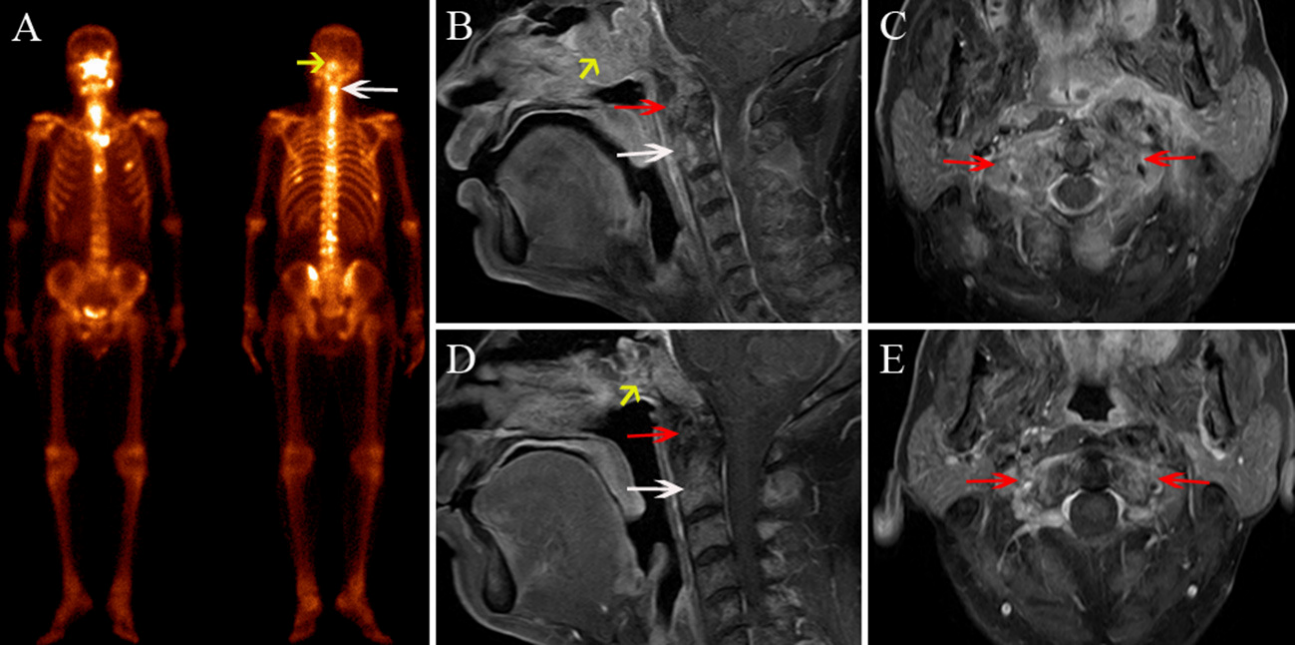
A 53-year-old male diagnosed with skull base recurrence combined with cervical spine metastasis after RT for NPC. (A) BS shows increased radiotracer uptake in the skull base (yellow arrow) and C2 (white arrow), multiple ribs, thoracic vertebrae, and lumbar vertebrae, also show increased radiotracer uptake. (B) Sagittal contrast-enhanced T1-weighted imaging shows an enhanced soft-tissue mass in the skull base (yellow arrow), C1 (red arrow), and C2 (white arrow). (C) Axial contrast-enhanced T1-weighted image shows enhanced soft-tissue mass and bone destruction in the bilateral aspect of C1 (red arrow). (D,E) In an MRI follow-up 3 months after RT, the sagittal enhanced T1-weighted image (D) and axial enhanced T1-weighted image (E) show that the lesion area has shrunken and the enhancement has declined.
